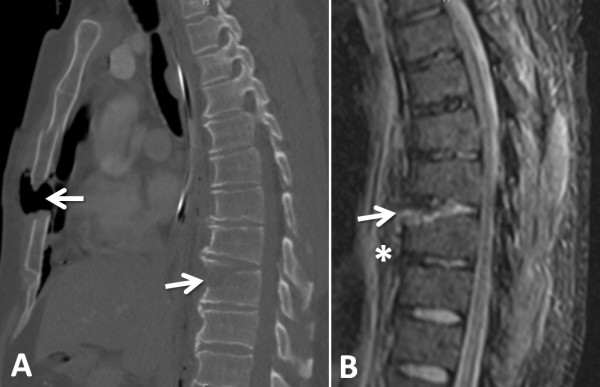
Sagittal CT scan (A) and STIR sequence in MRI (B) of the T9 hyperextension injury (arrows). The asterisk in panel B alludes to the extensive prevertebral hematoma.
